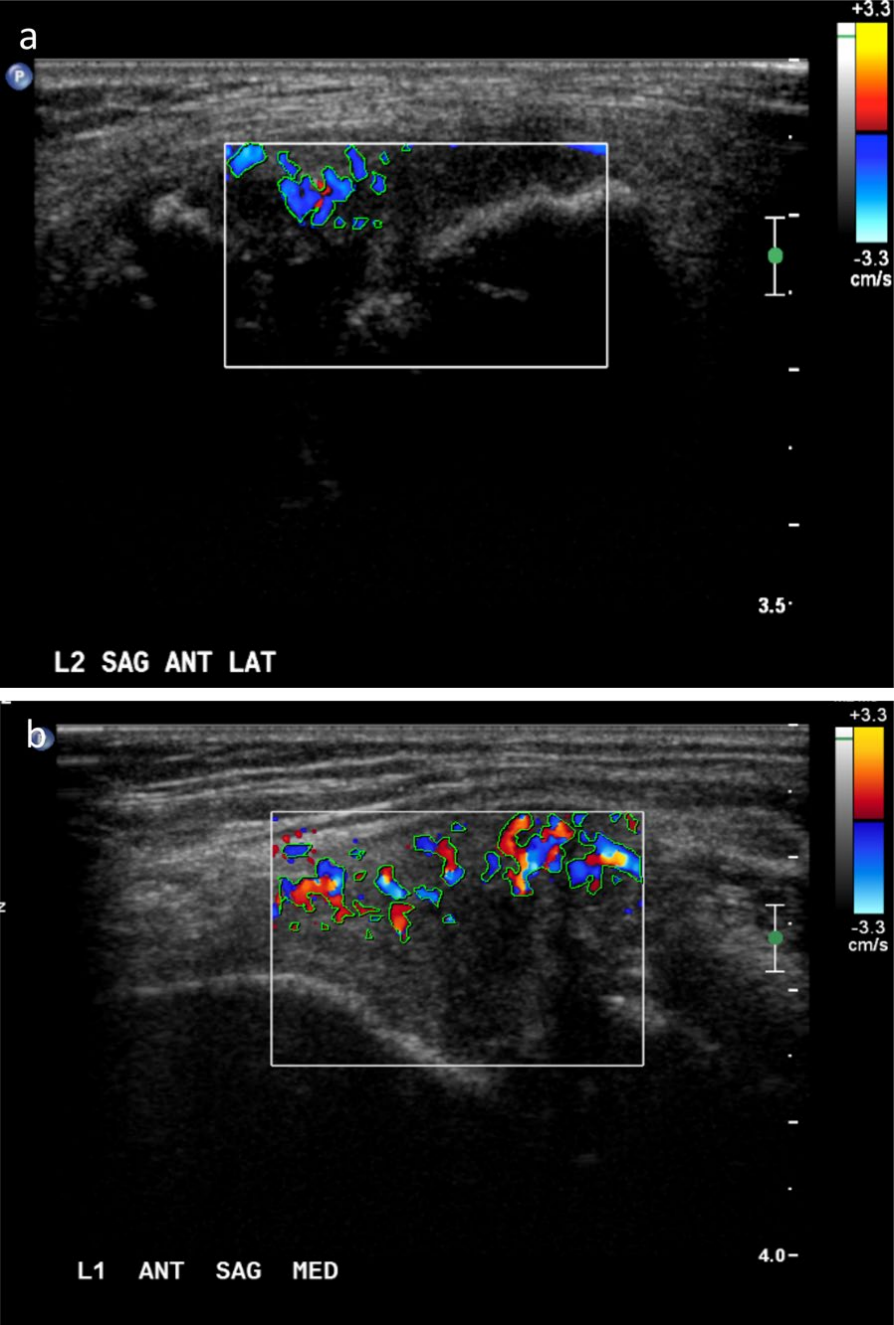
Increased synovial vascularity of joints of patients with hemophilic arthropathy on color Doppler ultrasound. a Eight-year old boy with severe hemophilia A and a history of prior bleeding into his right ankle. Mild hypervascularity is seen in his joint on color Doppler ultrasound. Color Doppler sonogram obtained on the sagittal anterior-lateral plane at the L2 level shows more than 4 color pixel dots encompassing less than 50% of the synovium extent within the color Doppler box which represents some degree of synovitis. Score equals 1. The area of color pixels within the color box is 4,257 units^2^. b Twelve-year old boy with severe hemophilia A and a history of recurrent bleeding into his left elbow. Extensive hypervascularity is seen in his joint on color Doppler ultrasound. Color Doppler sonogram obtained on the sagittal anterior medial plane at the L1 level shows more than 4 color pixel dots encompassing 50% or more of the synovium extent within the color Doppler box. Score equals 2. The area of color pixels within the color box is 13,605 units^2^
